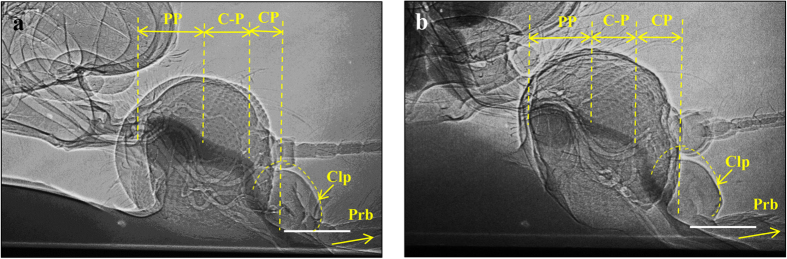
2D X-ray images showing the sagittal view of the head of a mosquito sucking a dilute iodine solution. The expansion state of the CP, C-P, and PP of *An. sinensis* (a) and of *Ae. togoi* (b). Scale bar indicates 200 μm.
